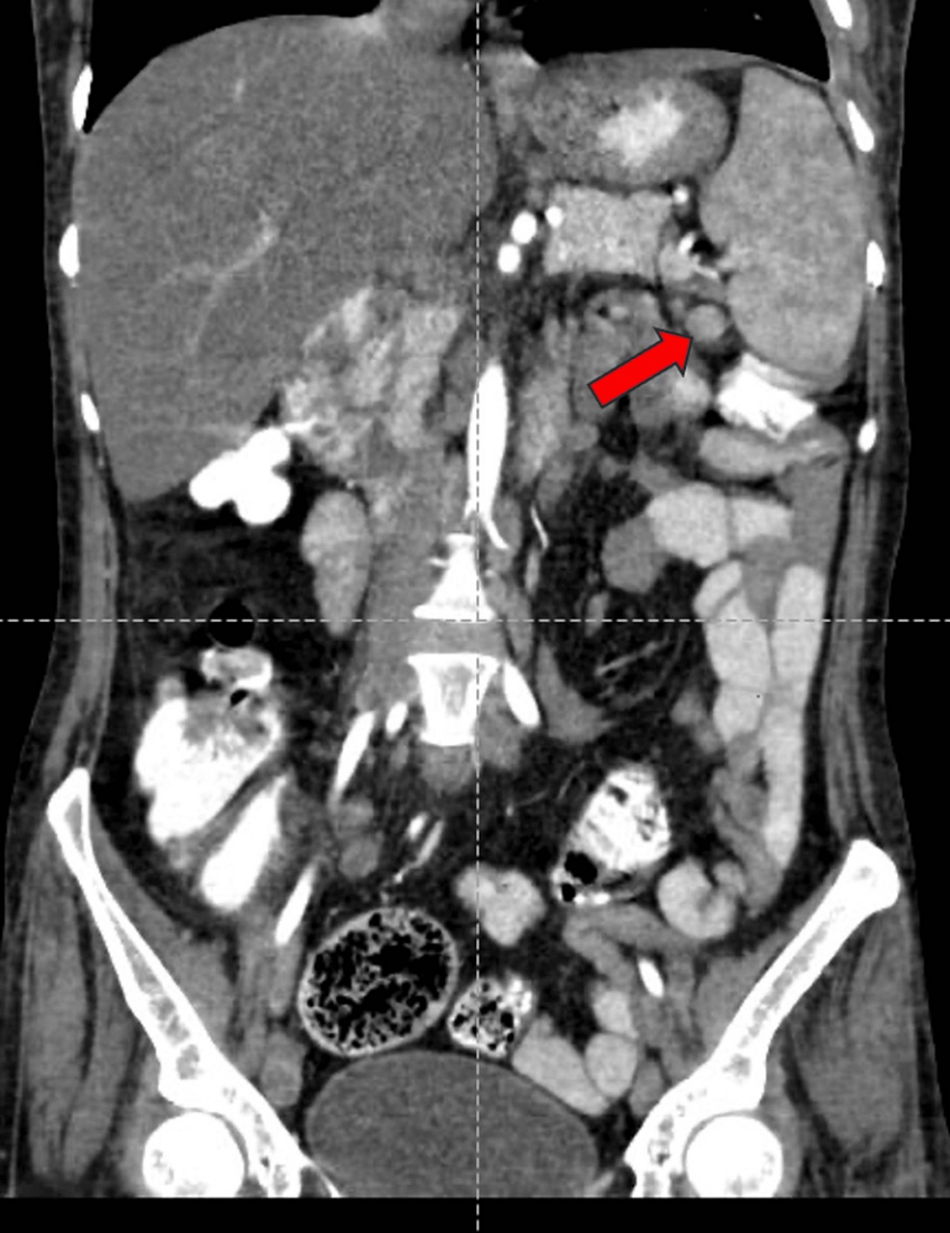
CECT-AP reformatted coronal section showing an enhancing lymph node at the splenic hilum shown by the red arrow. CECT-AP: contrast-enhanced computed tomography of the abdomen and pelvis
